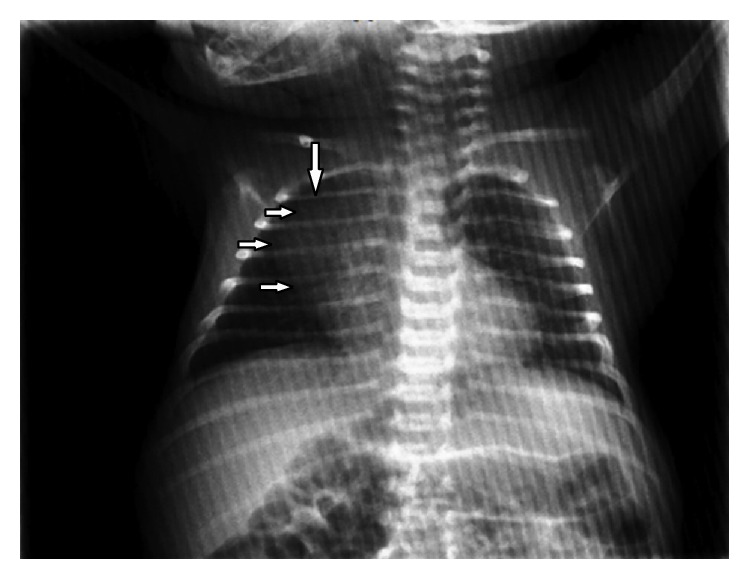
Enlargement of the cardiac silhouette in the right atrial region in posterior anterior chest radiography (marked with arrows).
